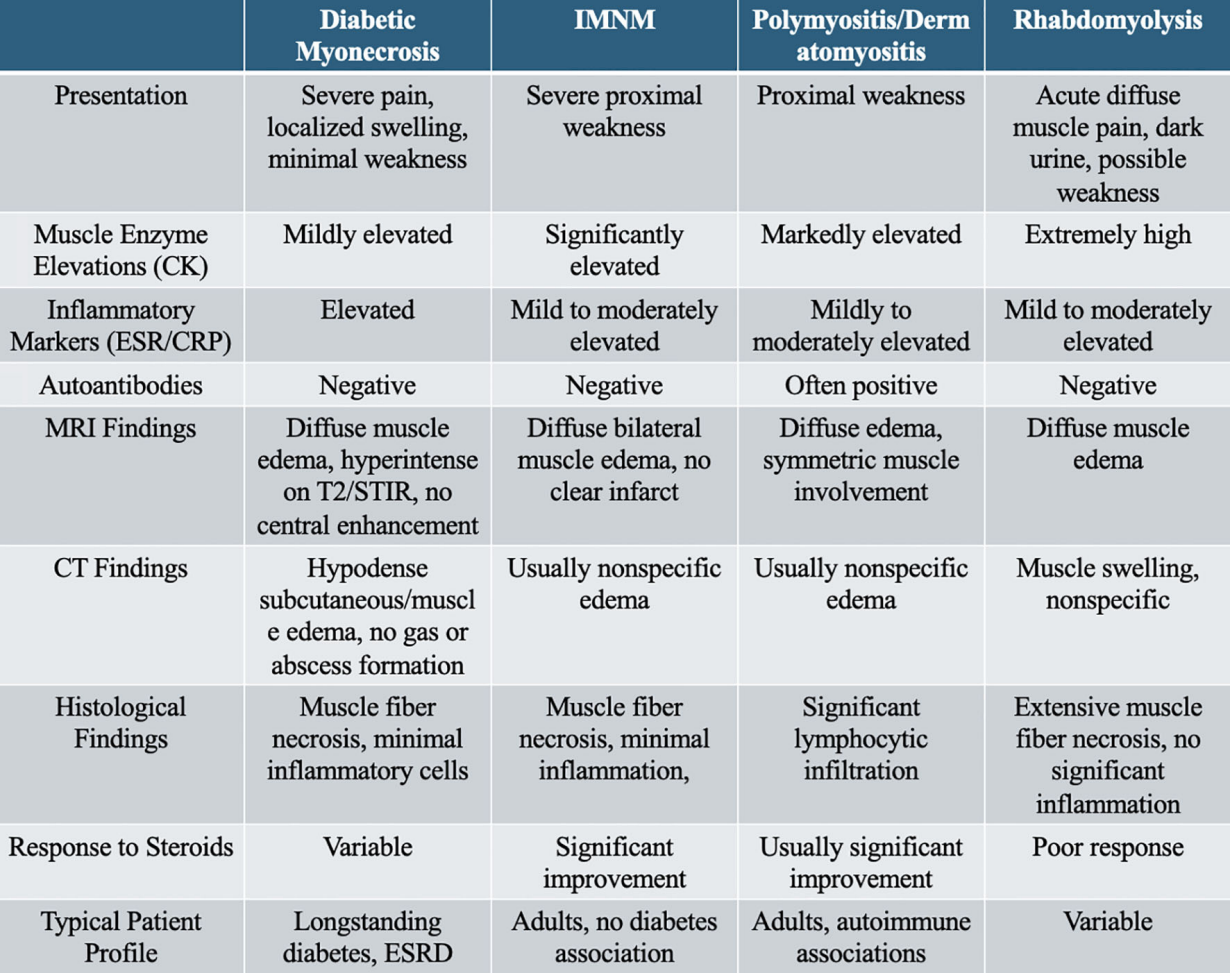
Key features between common pathologies. *CK*, creatine kinase; *ESR*, erythrocyte sedimentation rate; *CRP*, C-reactive protein; *IMNM*, immune-mediated necrotizing myopathy; *MRI*, magnetic resonance imaging; *T2/STIR*, T2-weighted/short tau inversion recovery.
